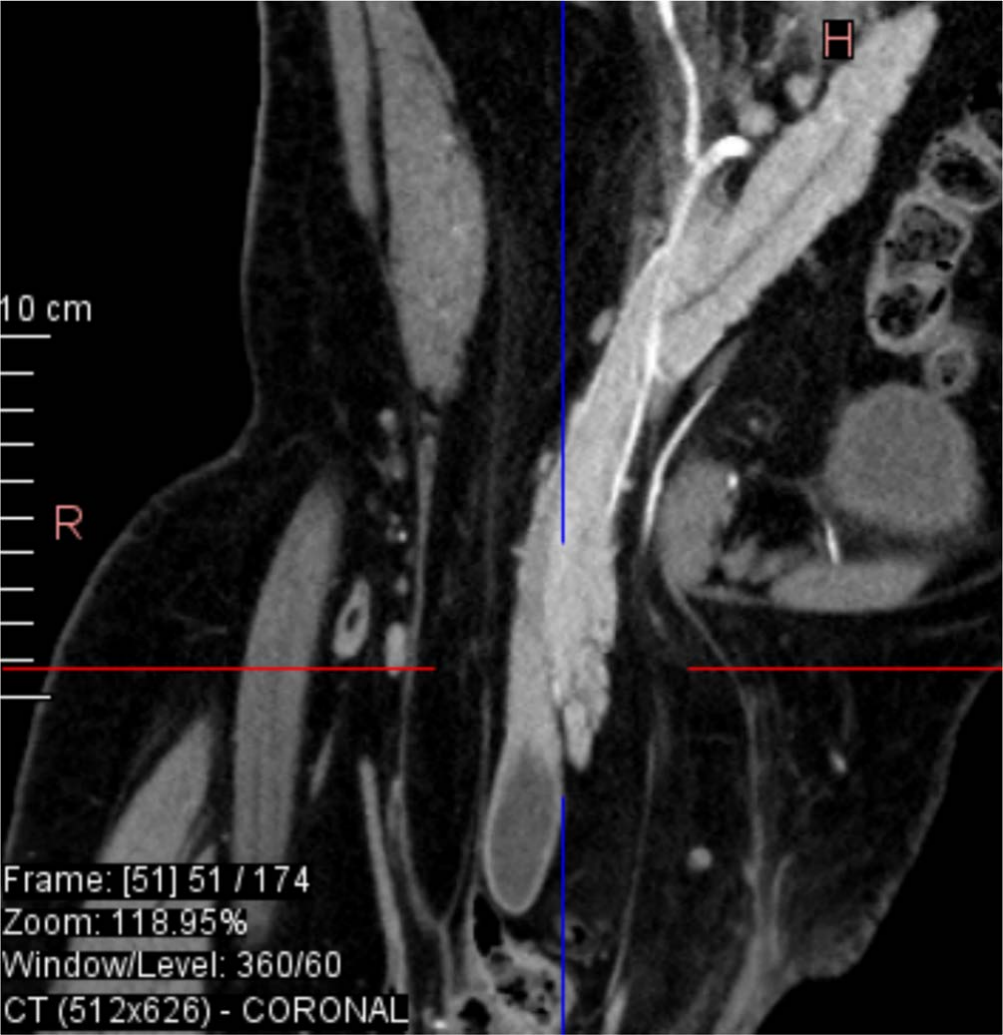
Coronal CT view visualization of vascularization of pancreatic head after i.v. contrast.
